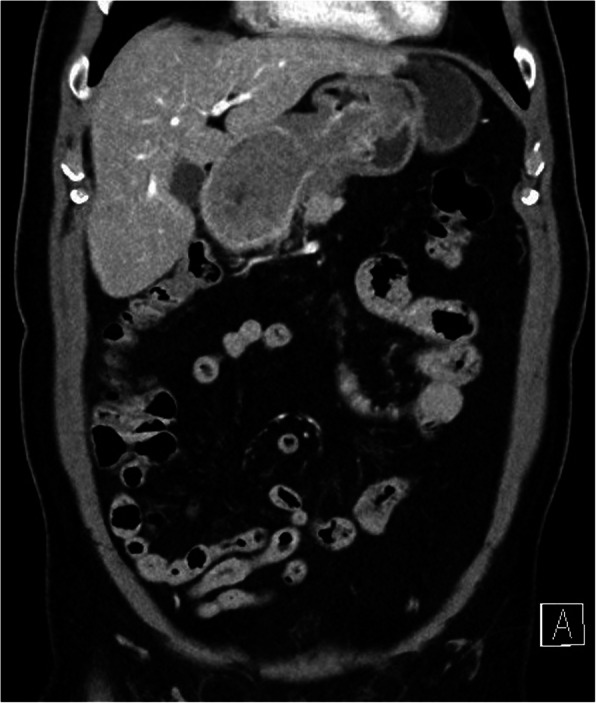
A CT scan demonstrating an intraluminal tumor of the lesser curvature of the gastric body producing a gastroduodenal intussusception with gastric outlet obstruction
